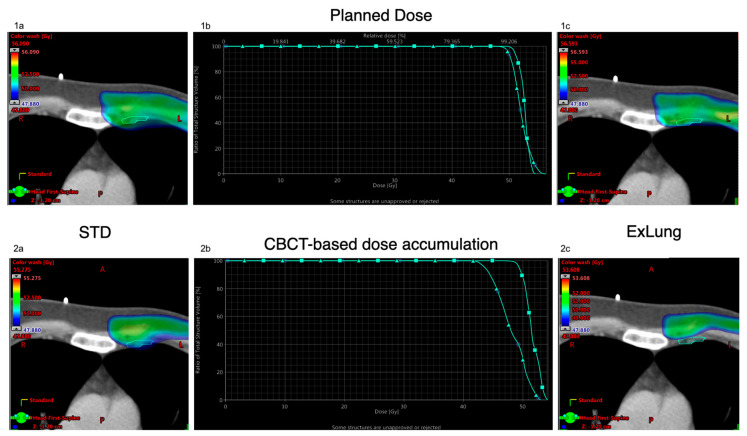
Impact of excluding lung tissue from the PTV. Delineation of the “planned” dose in the planning CT (1a–c) and the accumulated dose based on daily CBCT (2a–c) for the standard treatment plan using a 5 mm margin around the IMN-CTV (STD: 1a,2a)) and for the a treatment plan with a 5 mm planning treatment volume (PTV) margin excluding overlapping lung tissue (ExLung: 1c,2c).
